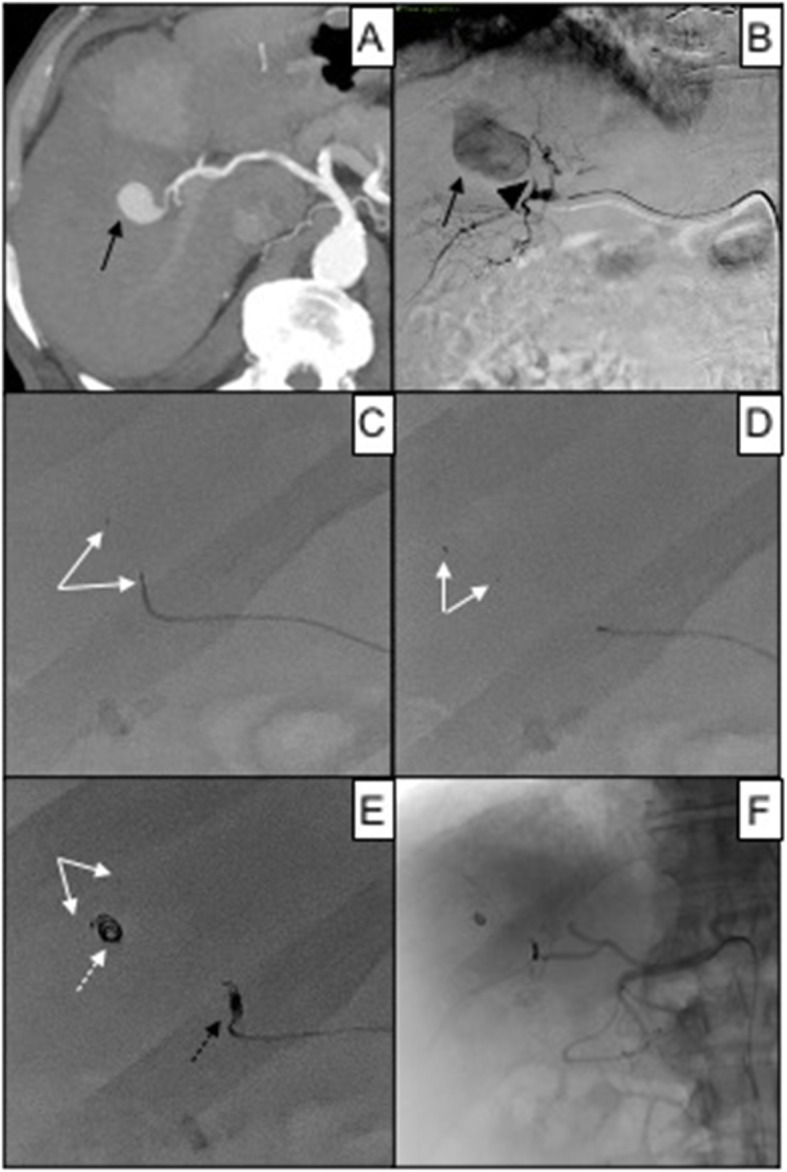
83 years old female affected by iatrogenic pseudoaneurysm of the right hepatic artery after RFA for HCC. In A, a contrast-enhanced CT scan in arterial phase shows the intraparenchymal vascular lesion (black arrow); in B, superselective DSA of the right hepatic artery confirms pseudoaneurysm (black arrow) refurnished by a short arterial feeder (black arrowhead); in C, attempt to perform MVP-3 (white arrows) embolization, target vessel caliper 1.1 mm; in D, due the shortness of the arterial feeder, MVP-3 (white arrows) migrated into the pseudoaneurysm sac; in E, proper embolization was obtained with a 2 mm Concerto^®^ Medtronic controlled detachment coil (black dotted arrow), after that another pushable coils (white arrow) migrated into the pseudoaneurysm sac together with MVP (white arrow); in F, fluoroscopic control showing resolution of the pseudoaneurysm
